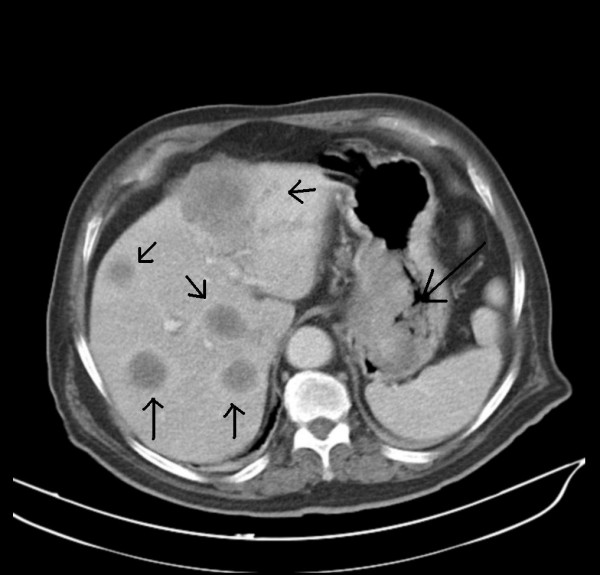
Computed tomography showing multiple solid lesions suggestive of metastases in both lobes of the liver, and a thickening of the medial wall-less curvature of the stomach and lymph node swelling around the gastro-hepatic ligament suspicious of gastric neoplasia.
